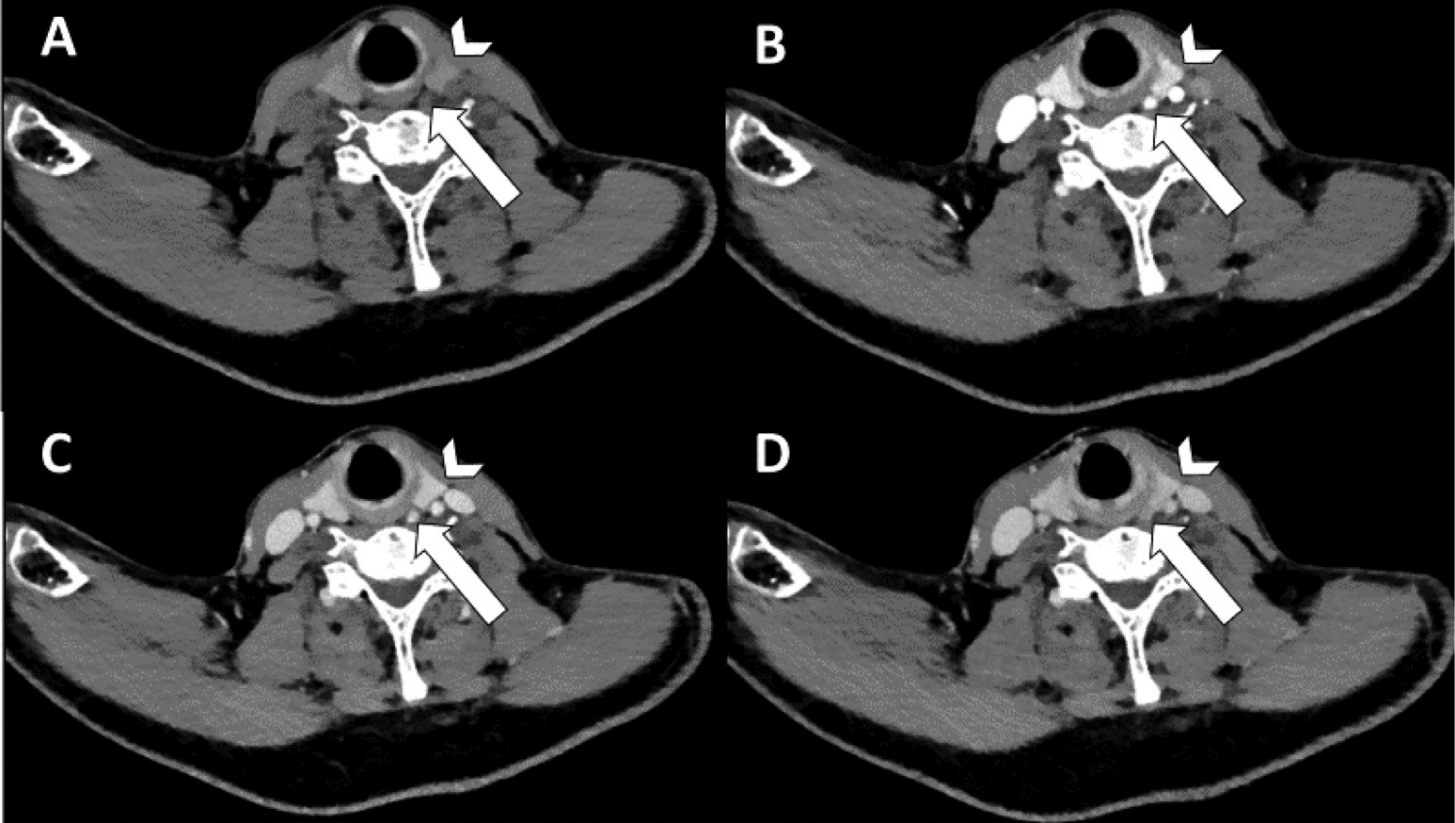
Four-dimensional CT with nonenhanced (A), arterial (B), venous (C) and delayed venous (D) phase of patient 1. The arrowhead is the thyroid gland. The arrow points to a lesion with lower attenuation than the thyroid gland on nonenhanced phase, with arterial enhancement and washout on the arterial and venous/delayed venous phases respectively. During surgery, after removing this lesion, PTH level did not decrease adequately, so further neck exploration was necessary.
